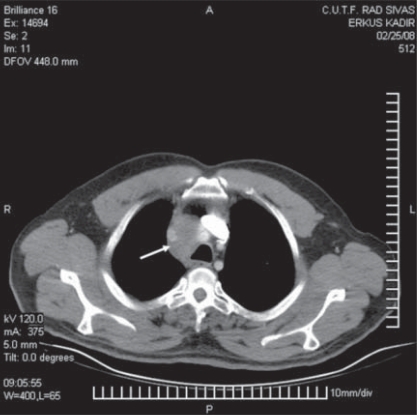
Panoramic radiograph of right lung shows (arrow) parathreacheal lymphadenopathy profile in the current case with non-small cell lung carcinoma
